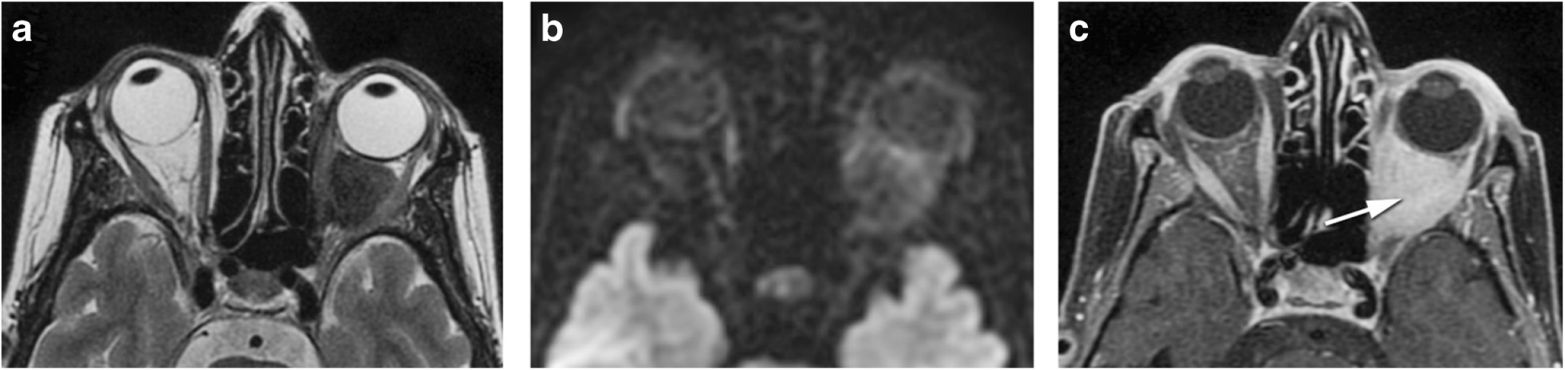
48-year-old female with idiopathic sclerosing orbital inflammation. Axial T2-WI (a), axial DWI (b) and enhanced axial T1-WI with fat signal suppression (c): large intraconal mass on the left (arrow), with no restriction diffusion, hypointense on T2, with marked enhancement after contrast. Notice enophthalmus, very unusual for a retrobulbar mass, together with absence of restriction diffusion, making it suspicious for sclerosing orbital inflammation
